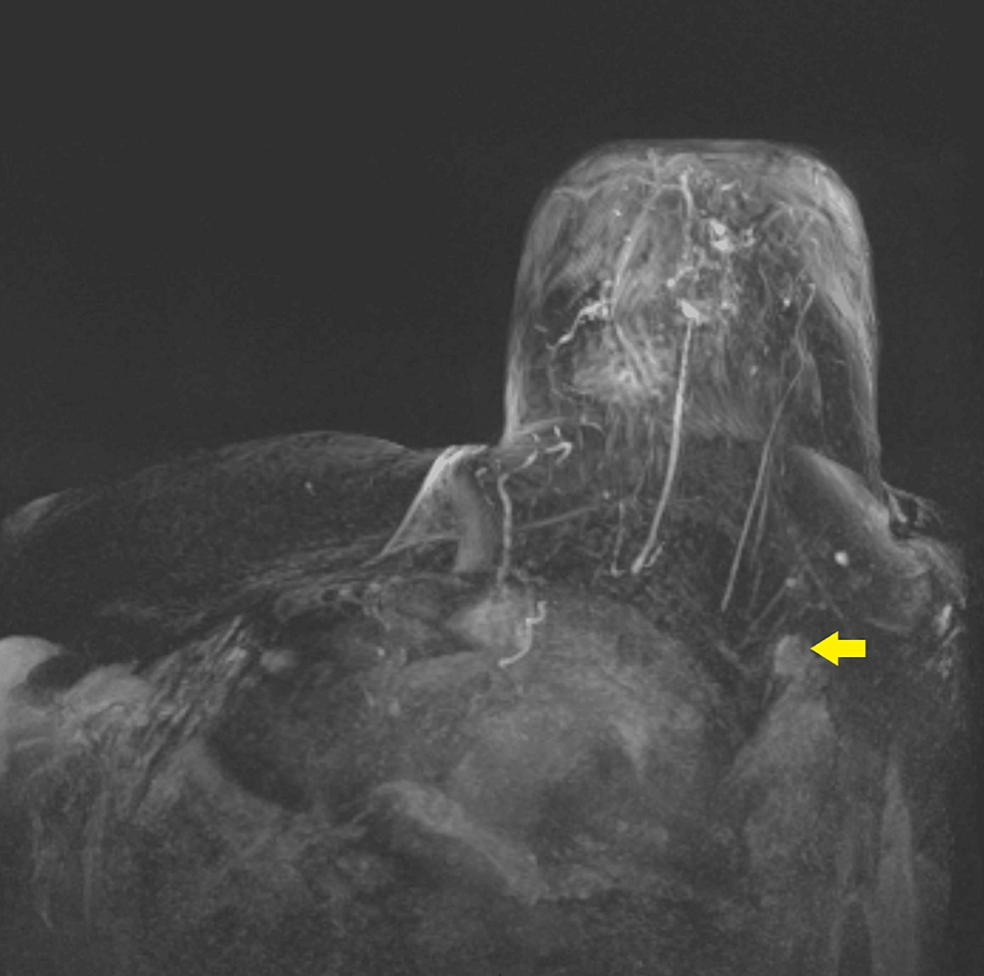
Two days post-ablation. Post-contrast dynamic MRI with subtraction MIP showing the absence of the primary lesion and a better outlined residual metastatic axillary lymph node (arrow) MIP: maximum intensity projection
